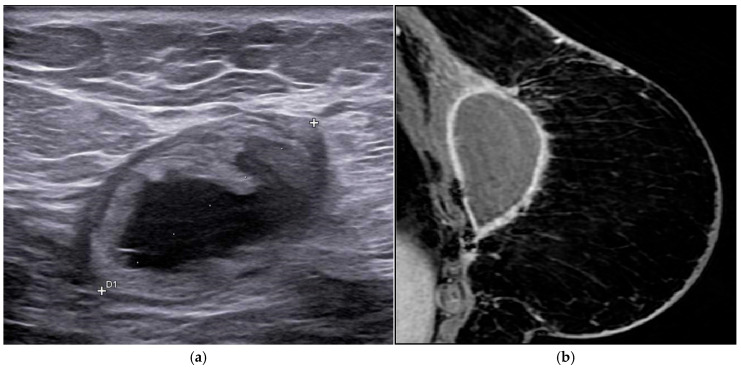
A 67-year-old woman, four months after fractionated radiotherapy, presents with a palpable sub-scar mass. Ultrasound evaluation shows a complex lesion with mixed solid–fluid components, without internal vascularity on color Doppler, consistent with an organizing fluid collection (a). MRI shows an extensive fluid collection with peripheral wall enhancement within the pectoralis muscle. The collection was partially drained and subsequently monitored with ultrasound follow-up, showing partial resolution over time (b).
